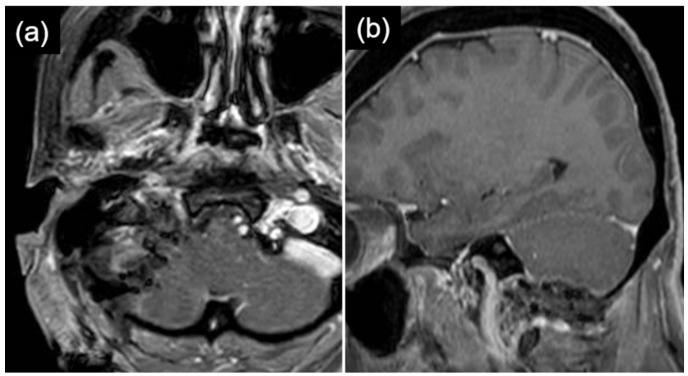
Postoperative contrast-enhanced T1-weighted magnetic resonance imaging demonstrating complete tumor resection. Axial (a) and sagittal (b) views are shown.
